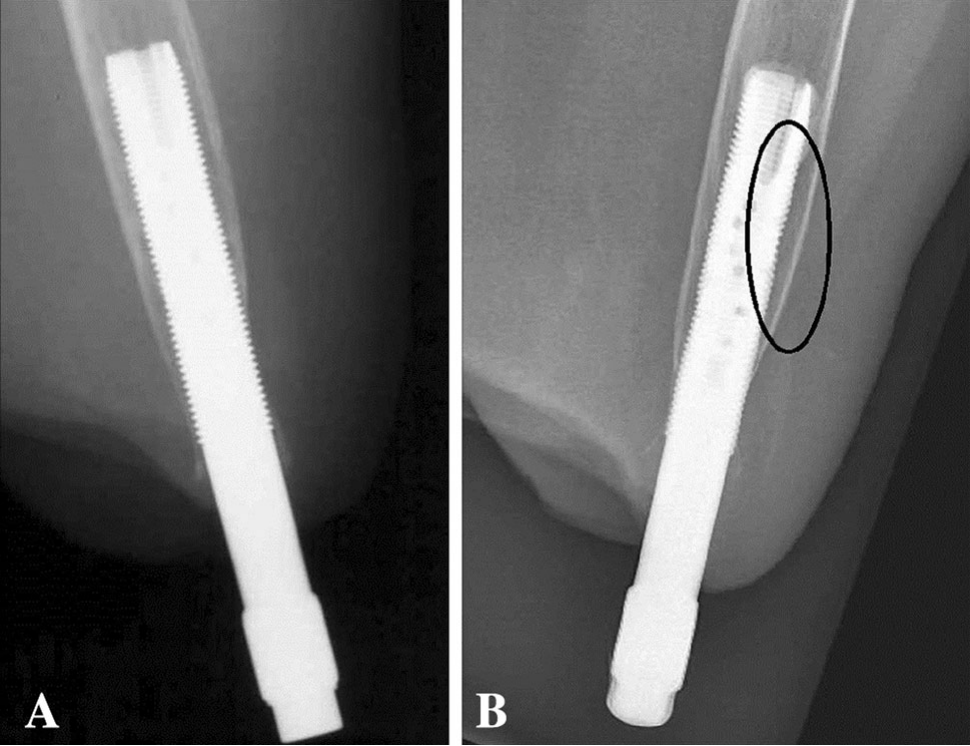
A postoperative radiograph at S2 is shown (A). Fifteen years later, cancellization is evident (B). This was one of the first patients who received an osseointegrated arm prosthesis. The fixture was custom-made and the technique of countersinking the fixture by 2 cm in the medullary cavity was not yet developed. This is why the distal bone looks as if it has already been resorbed at S2 (A).
